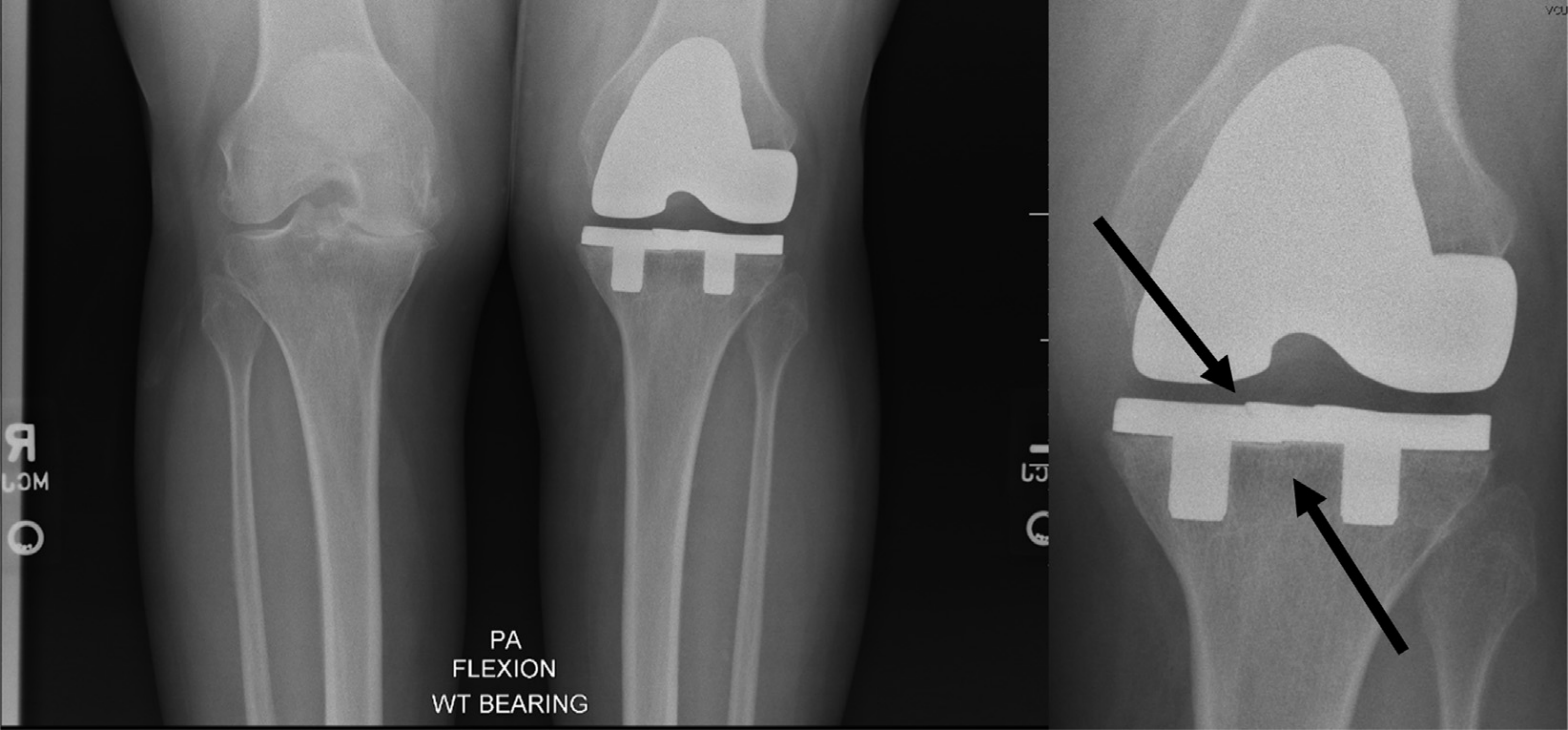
Standing posteroanterior flexion radiograph suggestive of tibial baseplate fracture (indicated by black arrows).
